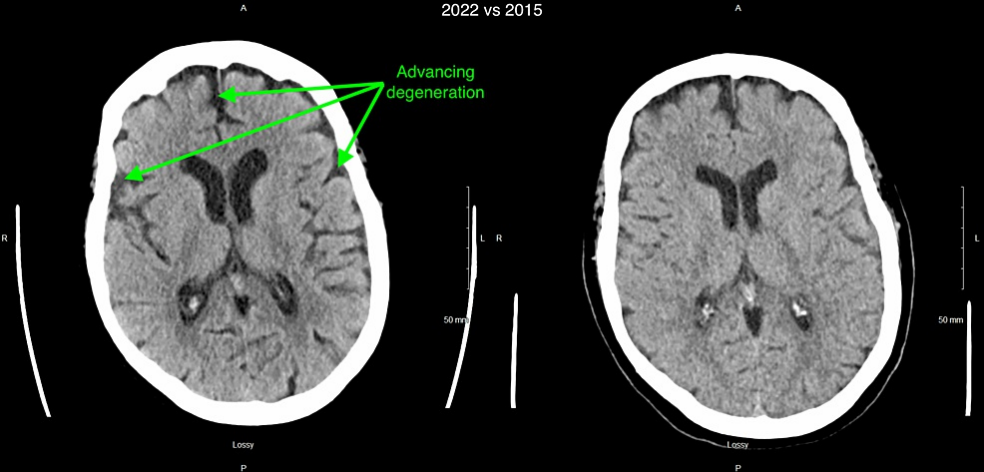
Computed tomography (CT) without contrast of the head 2022 vs 2015 (1) 2022 scan is on the left while 2015 scan is on the right
